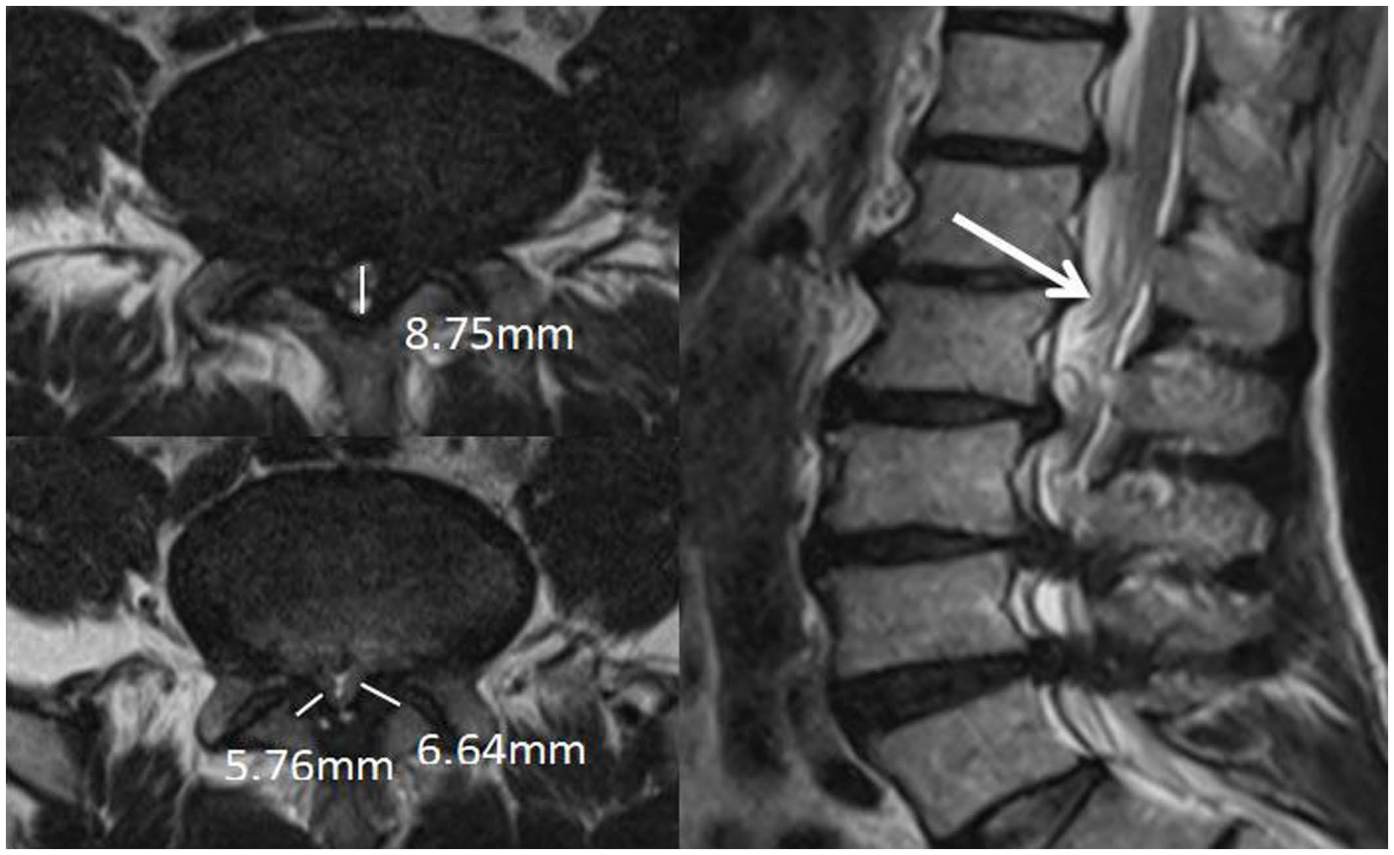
Patient, male, 67 years old, with recurrent low back pain for 2 years, recurrence and exacerbation for 3 months, lumbosacral pressure and percussion. Magnetic resonance imaging: bilateral ligamentum flavum hypertrophy and spinal stenosis, and the cauda equina nerve in the sagittal position at T2WI showed flaccid, tortuous, and entangled high-signal shadows.
